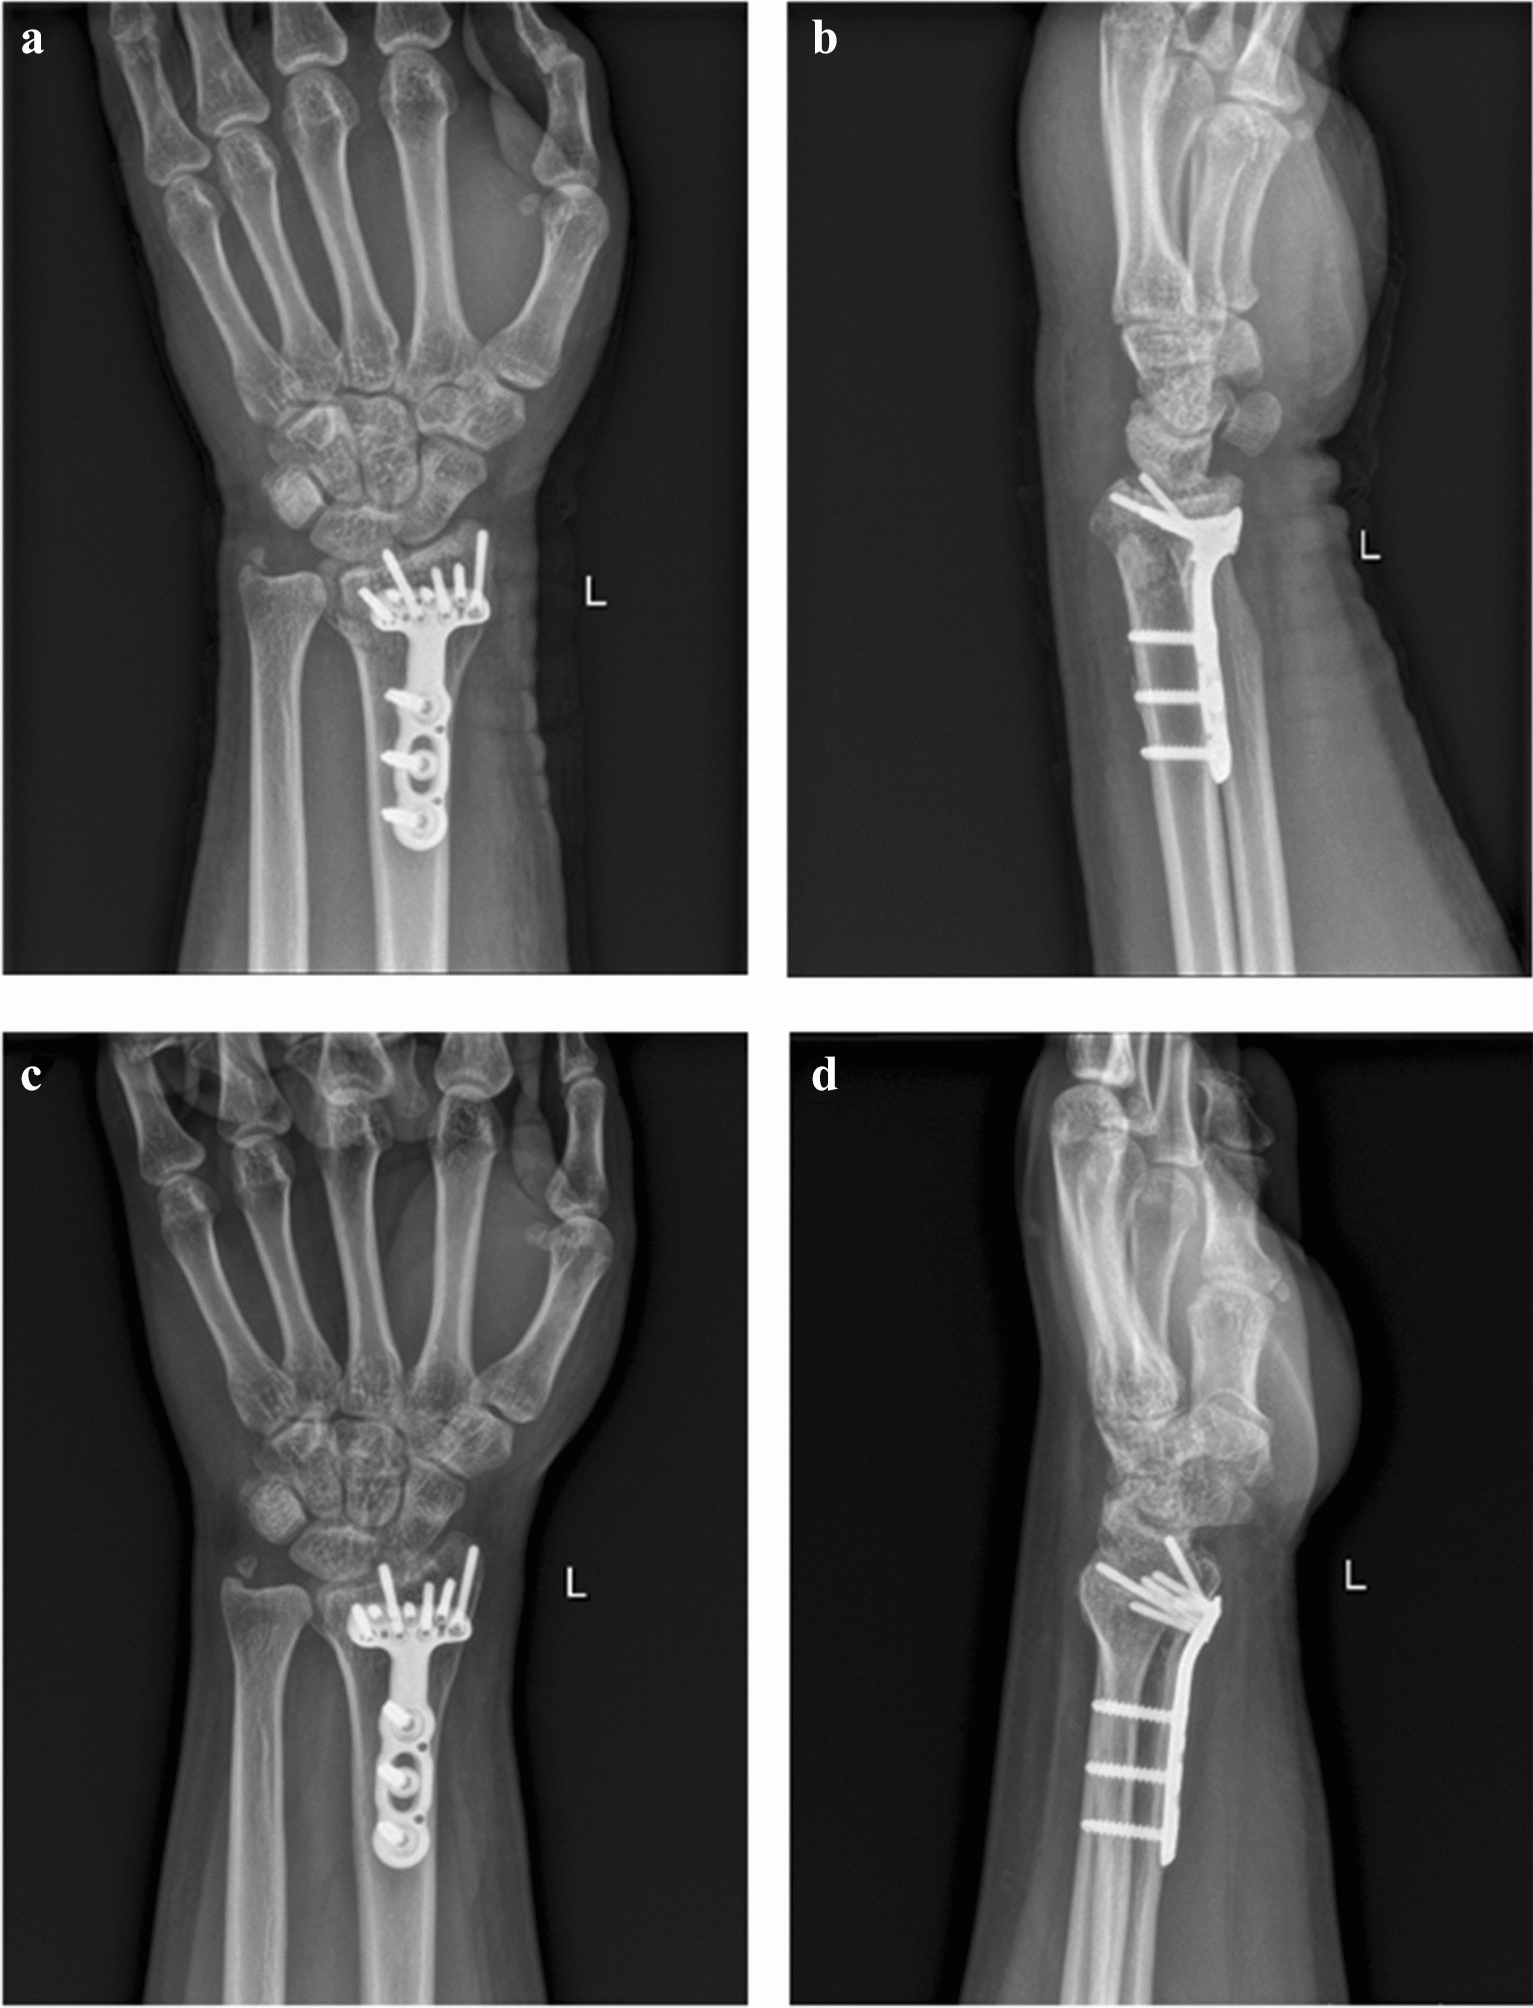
Radiographical images after the operation. a, b Anteroposterior (a) and lateral (b) radiographs showing that the alignment of the fracture and position of the plate were satisfactory on postoperative day 1. c, d Anteroposterior (c) and lateral (d) radiographs at 12-month post-operation, showing that the left wrist has completed bony union
